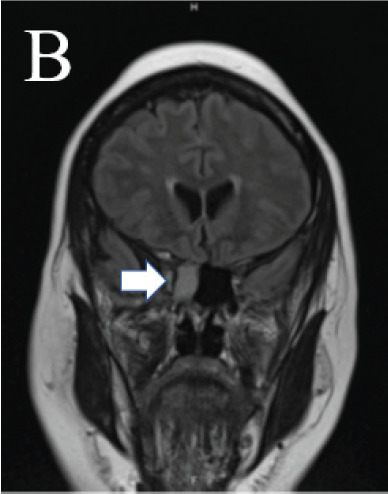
Coronal T1-weighted magnetic resonance imaging of the brain demonstrating herniation of the right inferior frontal lobe tissue through the right cribriform plate (arrow) into the sphenoid sinuses.
